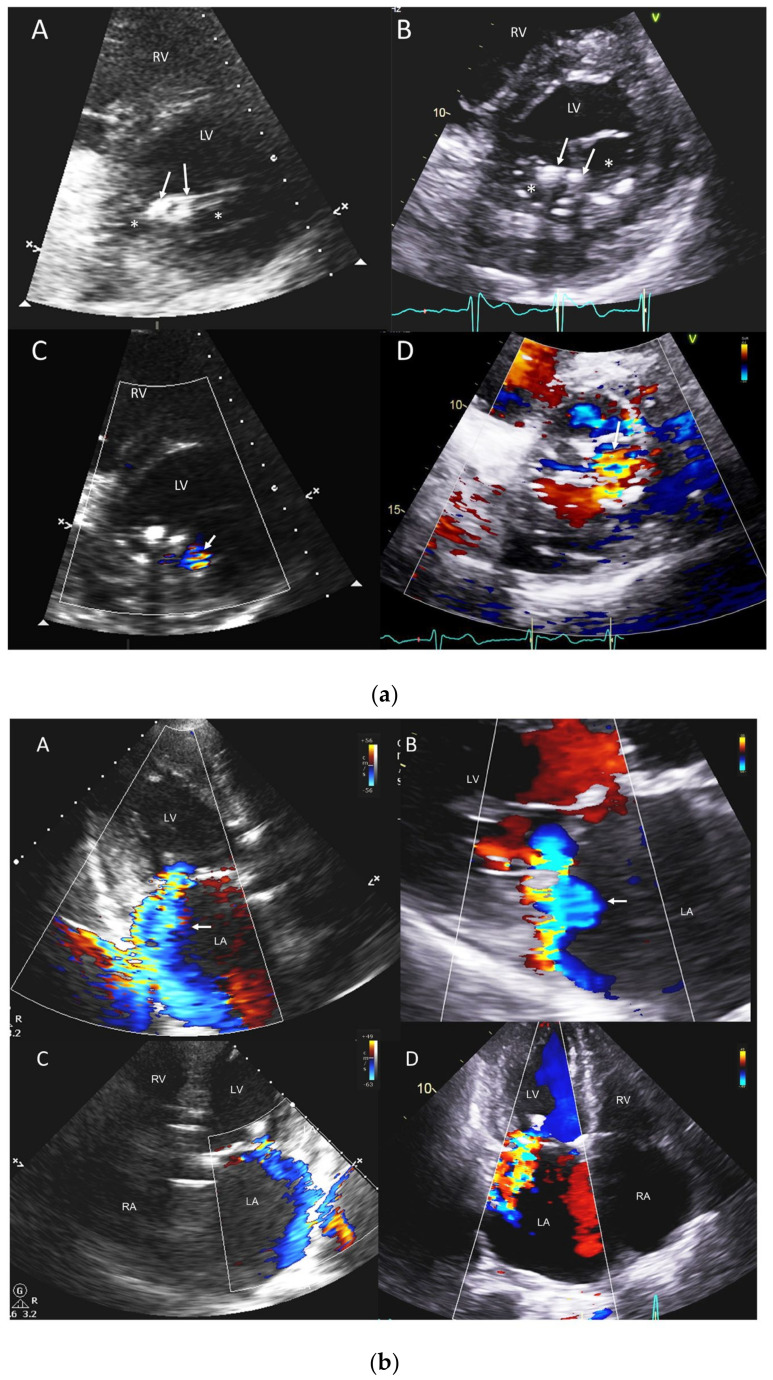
(a) POCUS in percutaneous transcatheter-based mitral valve repair. A 79-year-old male with residual MR post transcatheter edge-to-edge repair of the mitral valve (TEER) using mitral clips (2) implanted at an outside facility. POCUS images (left-side panels) compared to the sTTE images (right-side panels). (A,B) Parasternal short-axis views showing two mitral valve clips (white arrows (A,B)), a double-orifice mitral valve (white asterisks (A,B)); (C,D) color Doppler views at the same level showing origin of MR jet from the anterolateral orifice (white arrows (C,D)). (b) POCUS in percutaneous transcatheter-based mitral valve repair. POCUS sagittal color Doppler images (left-side panels) compared to the sTTE images (right-side panels) in the same patient as in Figure 1, showing significant residual MR post TEER. (A,B) Apical three-chamber and parasternal long-axis color Doppler views showing severe eccentric MR (white arrows (A,B)) hugging along the posterior wall (Coanda effect with the MR jet splaying circumferentially along part of the LA wall and shown in a single plane on 2D imaging, thereby underestimating the jet size) of a dilated LA. (C,D) Apical four-chamber views with LV on the right side on POCUS image (C) and on the left side on sTTE image (D), with moderate to severe appearing eccentric MR hugging the lateral wall (Coanda effect) of a severely enlarged LA. LA, left atrium; LV, left ventricle; RA, right atrium; RV, right ventricle.
